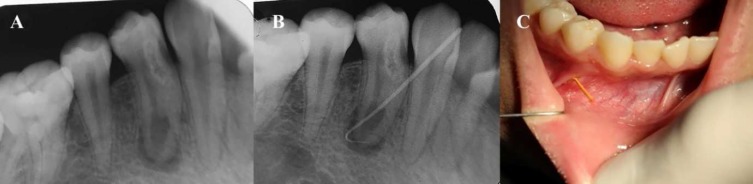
A) Preoperative periapical radiograph of the first mandibular premolar with chronic periapical pathosis; B) Periapical radiography of tracing of the sinus tract; C) Tracing of the sinus tract with #25 gutta-percha
